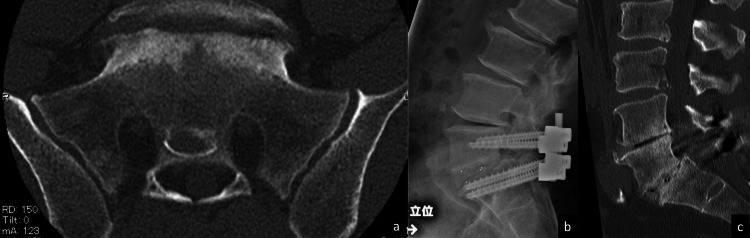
Case 1: A case without bony ankylosis of the sacroiliac joint. Bone fusion was achieved one year after surgery: (a) preoperative axial CT slice showed the absence of bony ankylosis; (b) postoperative X-ray during extension showed no sign of bone fusion failure around the endplates; and (c) postoperative sagittal CT showed bone continuity between L5 and S1 endplates.
